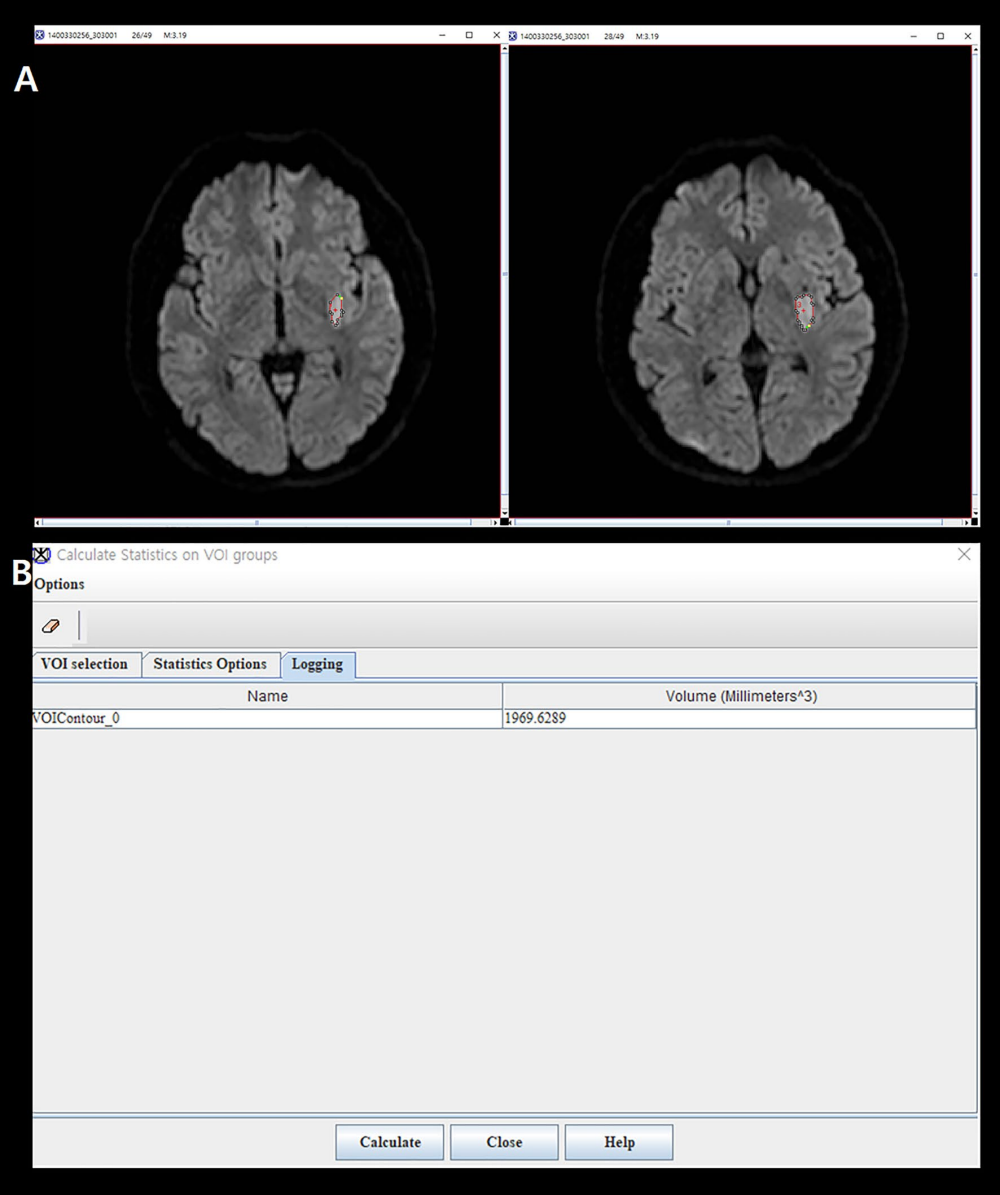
A representative example of how to calculate the infarct growth velocity. We quantitatively measured initial infarct volume using MIPAV version 11.0.0. program. The infarct lesions of each slice were delineated in a semi-automated way. After that, the program automatically calculated the volume by summing the lesion area of each slice. The initial infarct volume measured in this way was 1.97 mL, and MRI was conducted in this patient 1 h after symptom onset. Therefore, according to the formula, it is calculated that this patient had an IGV value of 1.97 mL/h.
